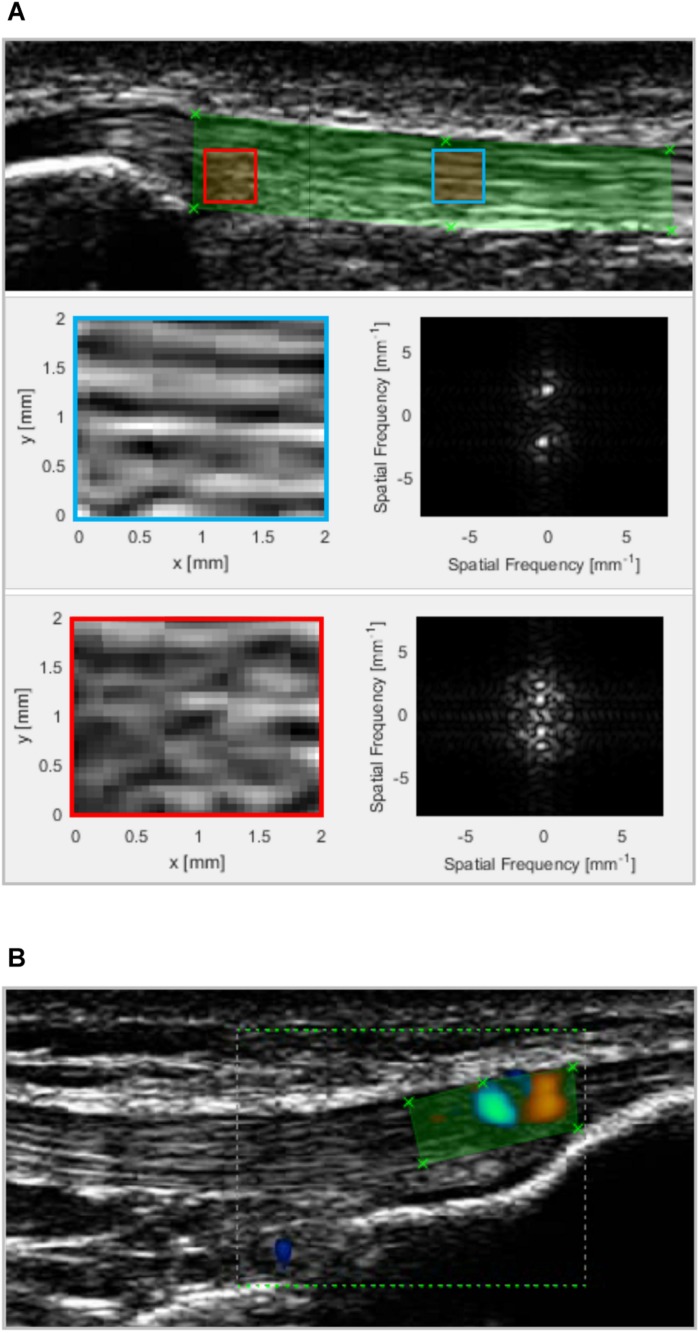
(A) Patellar tendon micromorphology was assessed by applying a spatial frequency analysis on ultrasound images obtained from the proximal (shown) and distal tendon. All possible 32 × 32 pixel kernels within a polygonal region of interest (ROI; green) were filtered and 2D fast Fourier transformed. The blue and red squares represent two kernels with a high and low degree of fascicle packing and alignment and, thus, peak spatial frequency (2.2 and 1.4 mm^–1^, respectively). The panels on the right to the enlarged kernels show the respective frequency spectrum. The average PSF value of all kernels was used in the statistical analysis. (B) Vascularisation was quantified by measuring the color area within a polygonal ROI that covered all intratendinous color doppler signals (exemplary distal image shown).
